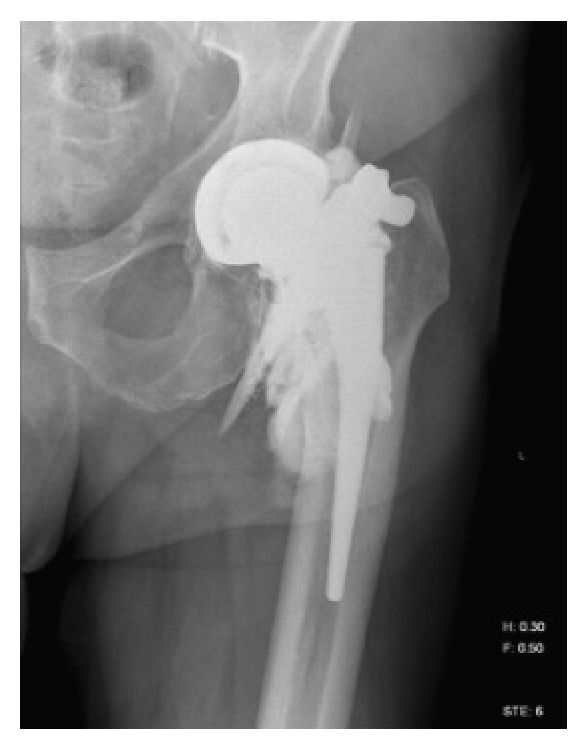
X-ray arthrogram showing mild contrast tracking along the proximal lateral aspect of the femoral component.
